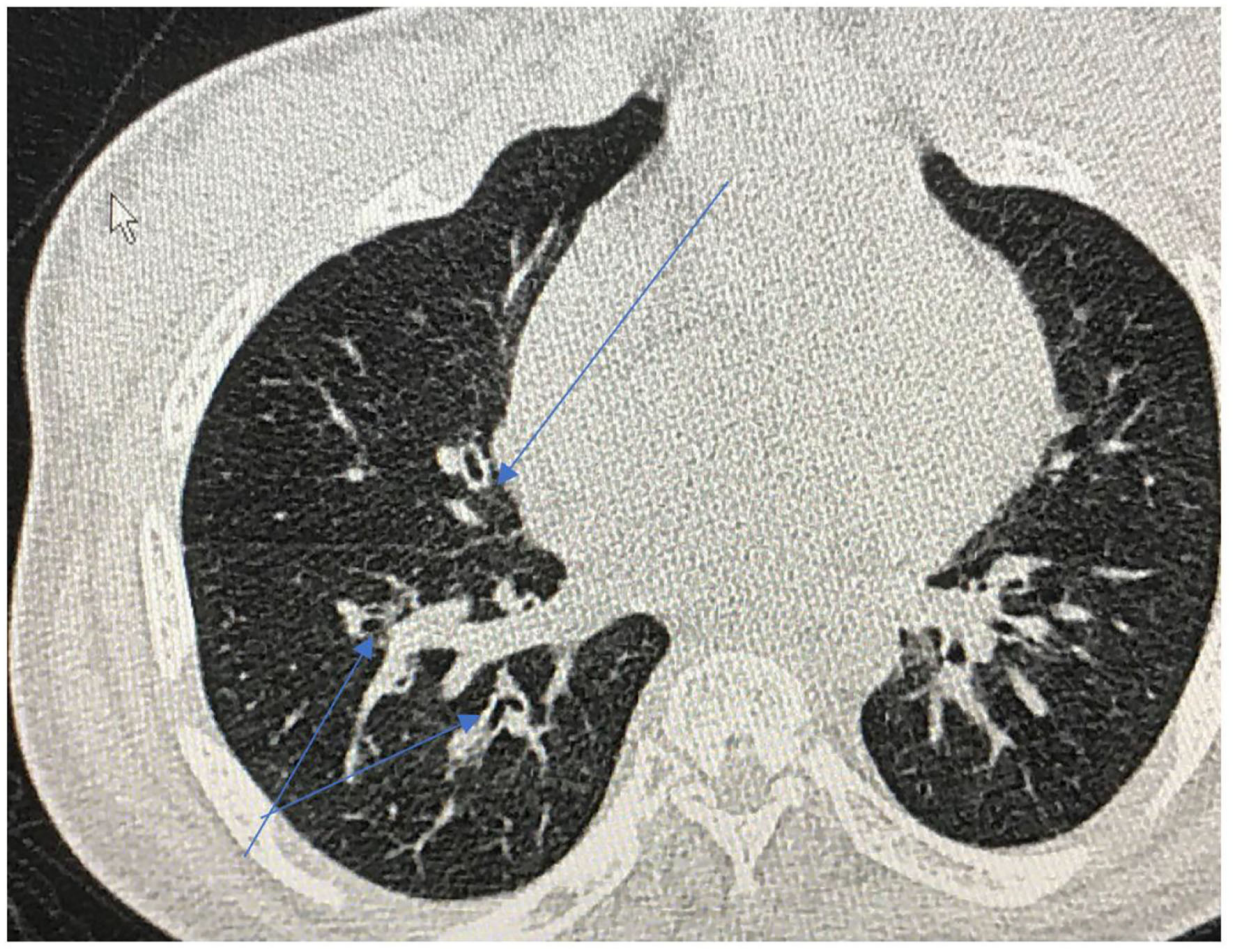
Cross sectional thoracic CT scan shows bronchial dilation and early bronchiectasis in adult MPS type IV.
